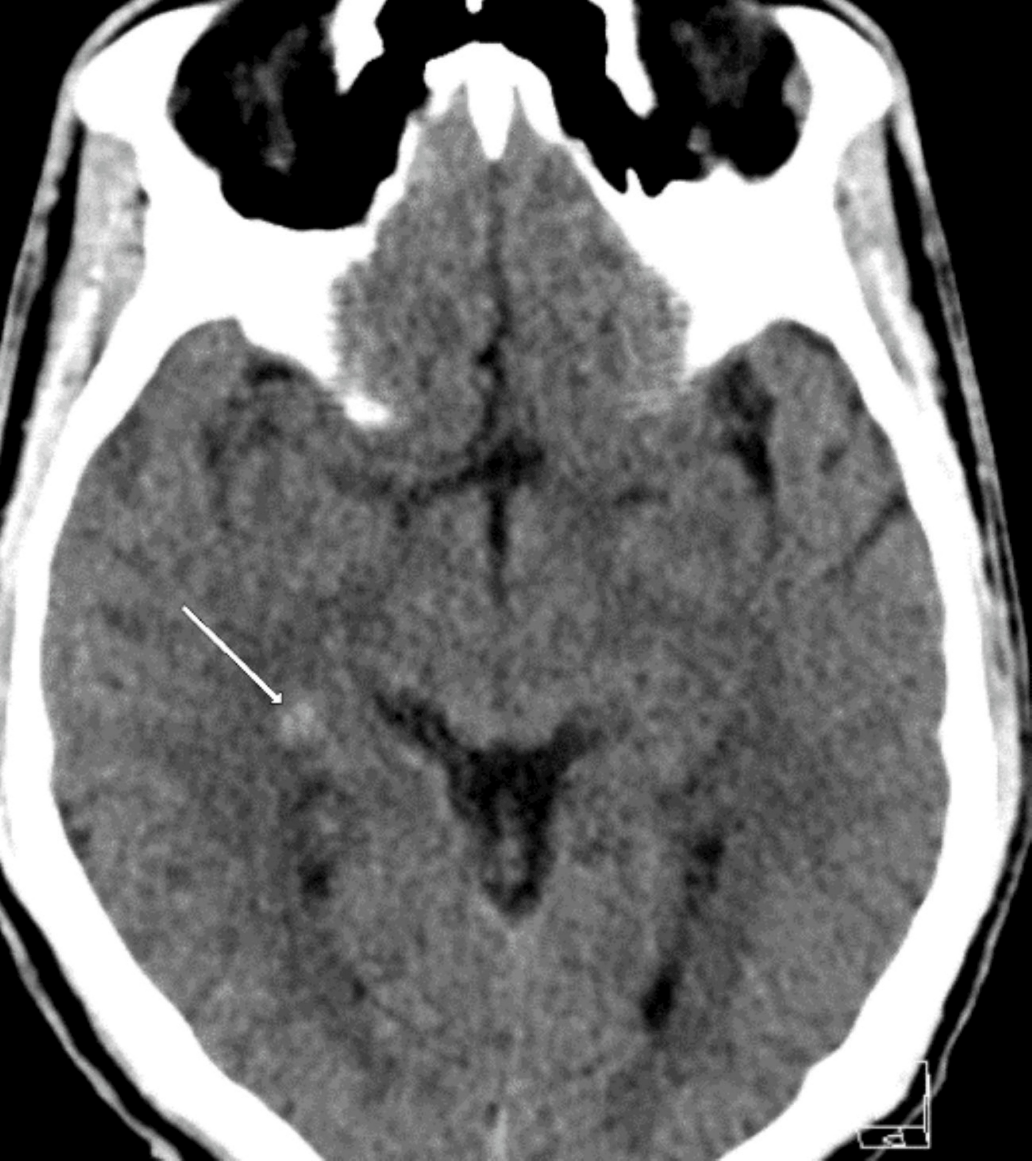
Axial non-contrast enhanced head CT with a small mildly hyperdense lesion adjacent to the right lateral ventricle at the medial right frontoparietal junction (arrow) CT: computed tomography
